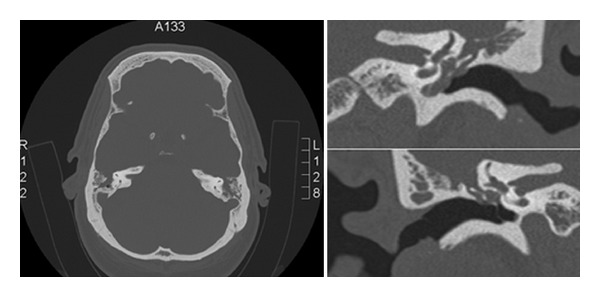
Axial and coronal CT images showing the tegmen defects.
